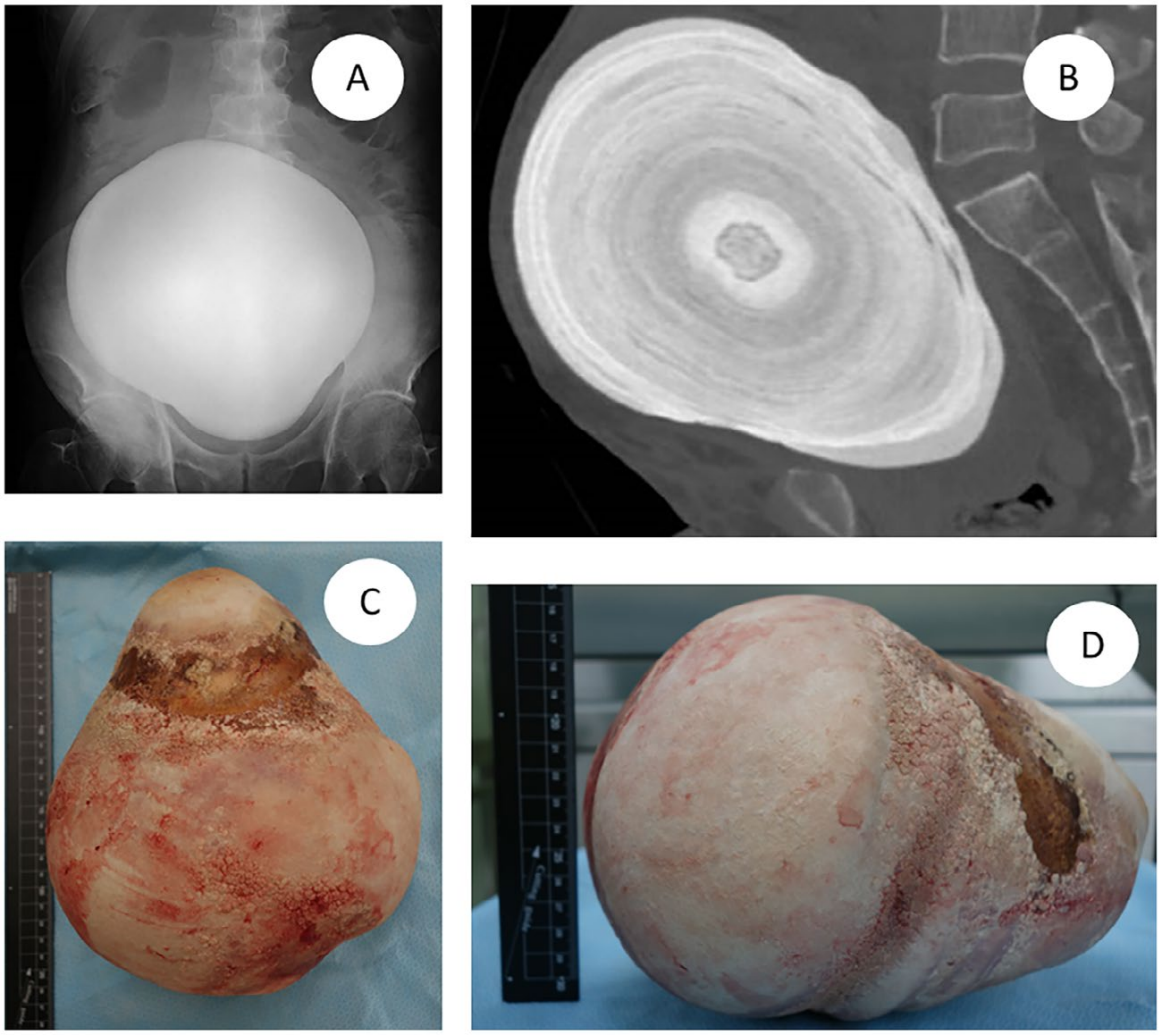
Neobladder stone in this case. (A) Abdominal X‐ray image. (B) Sagittal view of plain CT scans. (C, D) The removed stone.
